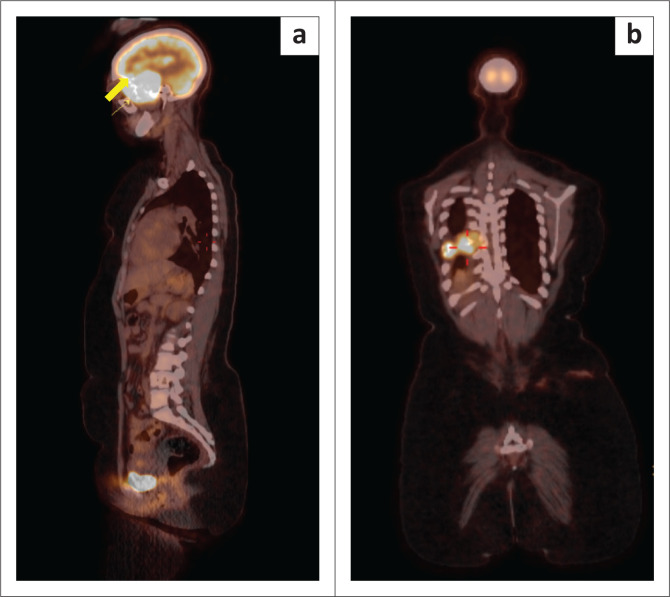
(a, b) ^18^F-FDG positron emission tomography (PET)/CT demonstrates the avid left skull-base tumour (yellow arrow) (a) and the avid focus in the right posterior 8th rib (red cross hairs) (b).
